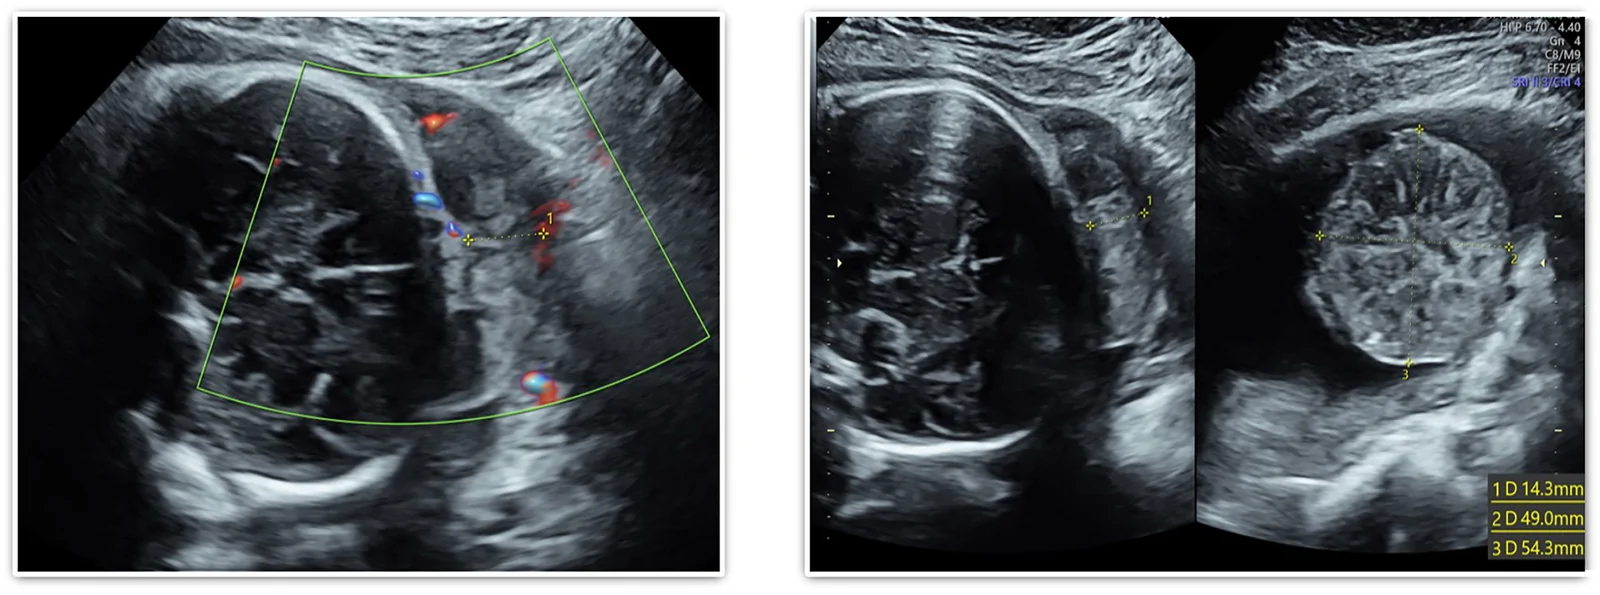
Ultrasound images of the glomovenous malformation (GVM) presenting as a mixed heterogeneous avascular mass in the fetal scalp located on top of the fetal head within the subcuticular tissue and separate to the bone.
